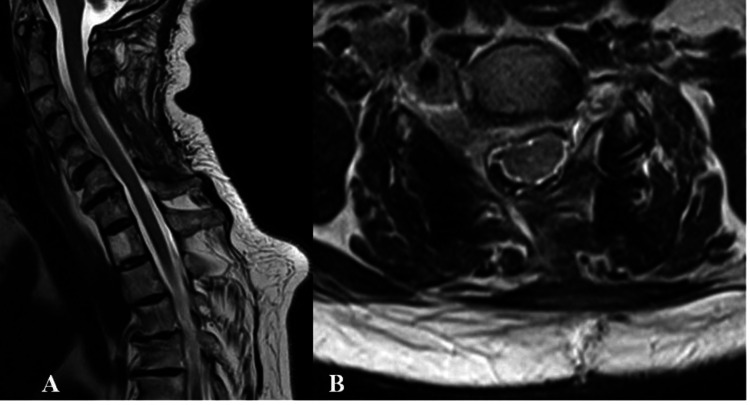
Follow-up cervical MRI after three months. Subtotal extirpation of the tumor lesion with adequate surgical decompression of the spinal cord. A: Sagittal projection with subtotal extirpation of the tumor lesion with adequate surgical decompression of the spinal cord. B: Axial projection with subtotal extirpation of the tumor lesion with adequate surgical decompression of the spinal cord.
